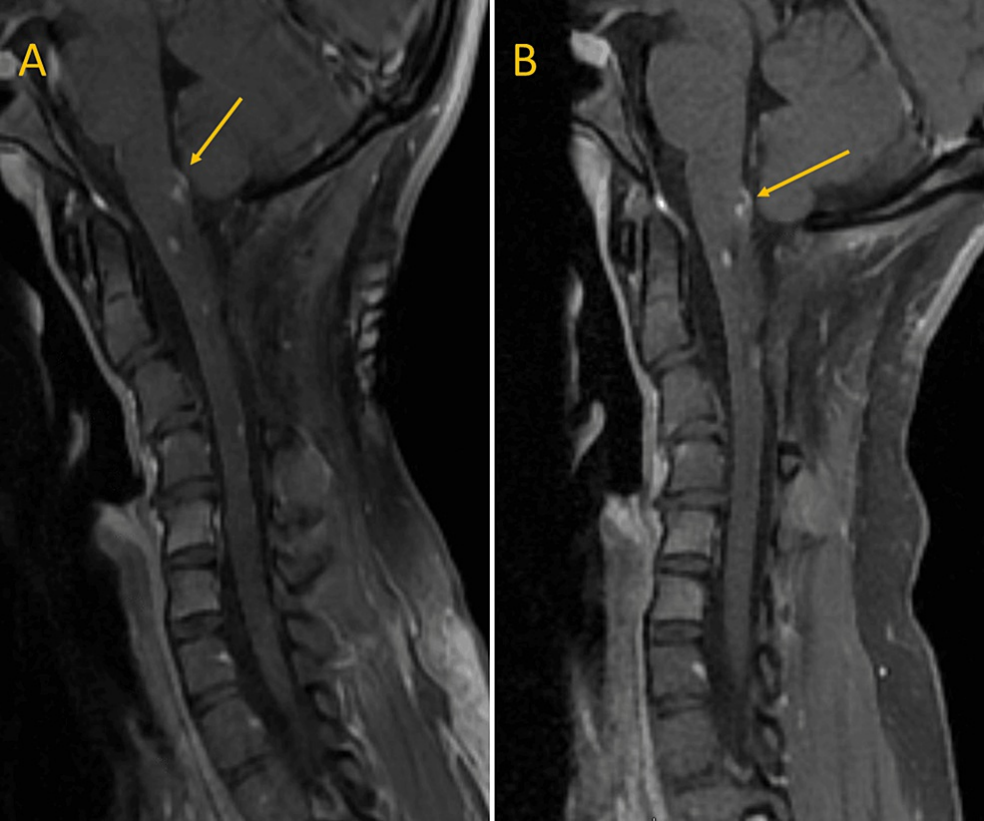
The 15- and 18-month follow-up magnetic resonance imaging (MRI) of the cervical spine (T1 post-contrast) (A) The 15-month follow-up MRI of the cervical spine. (B) An 18-month follow-up MRI of the cervical spine evidenced a sustained radiologic response.
